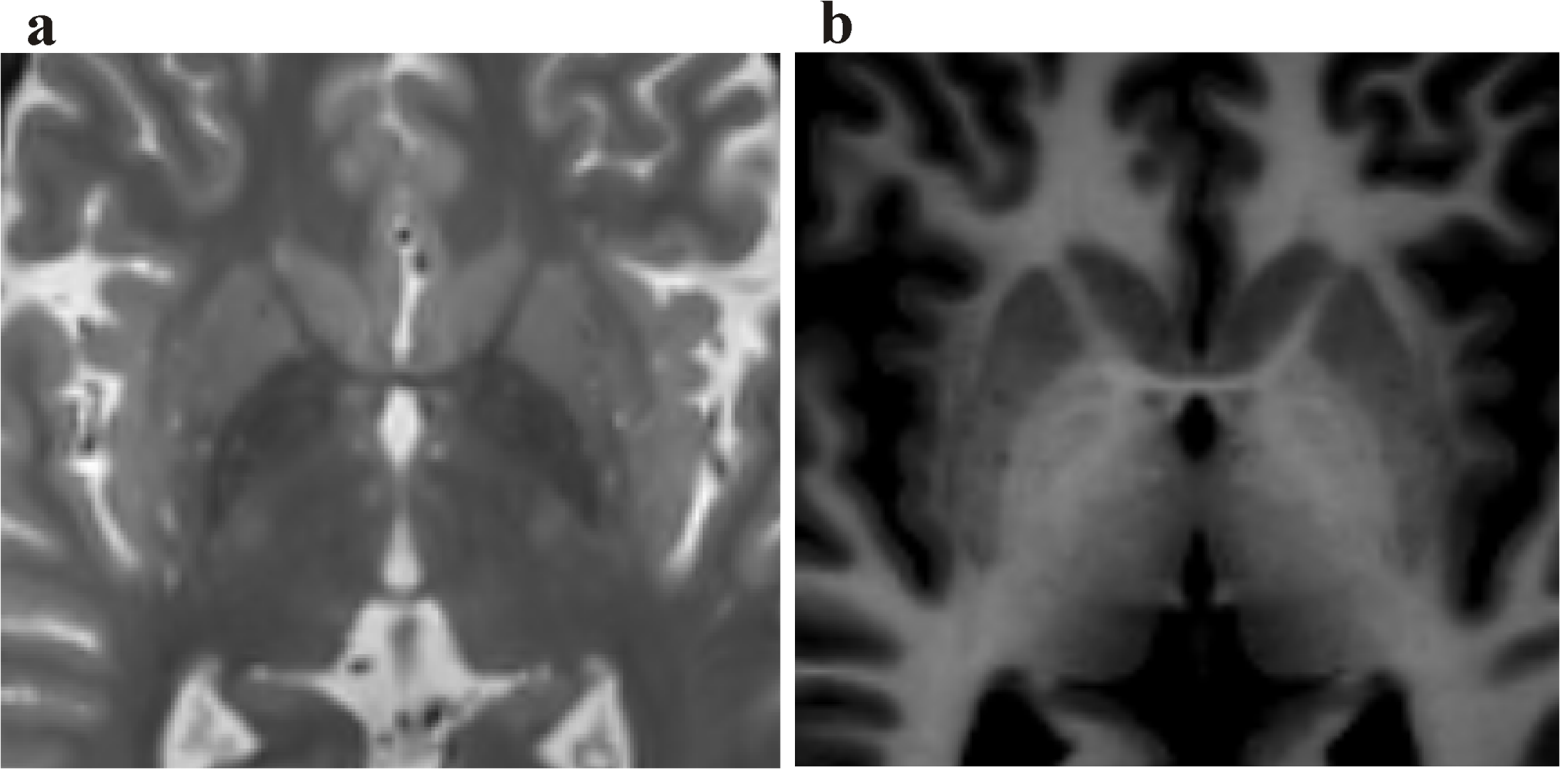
Representative axial T2-weighted (a) and MDEFT (b) sequence of a patient undergoing DBS implantation of the GPi. Note the good demarcation of the subnuclei of the basal ganglia on the MDEFT image.
